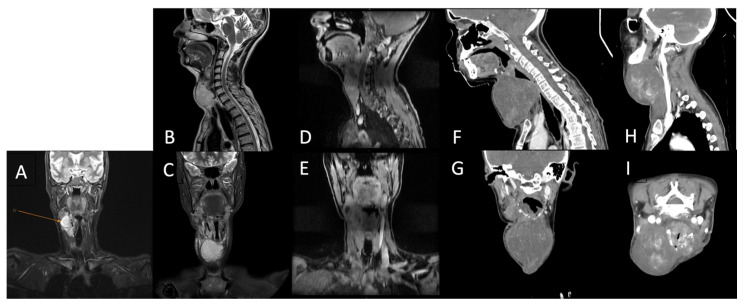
Cervical region using MRI (A)—After chemotherapy (5 months post-surgery); (B,C)—after adjuvant chemotherapy; (D,E)—after adjuvant radiotherapy; (F,G)—After first round of palliative chemotherapy; (H,I)—after second round of palliative chemotherapy.
